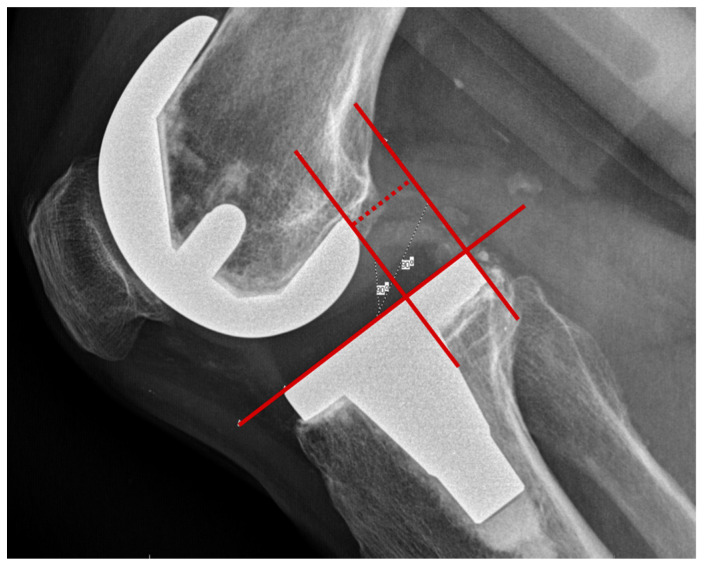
Measurement technique of the posterior tibial translation in stress radiographs in approximately the 90° posterior drawer position.
